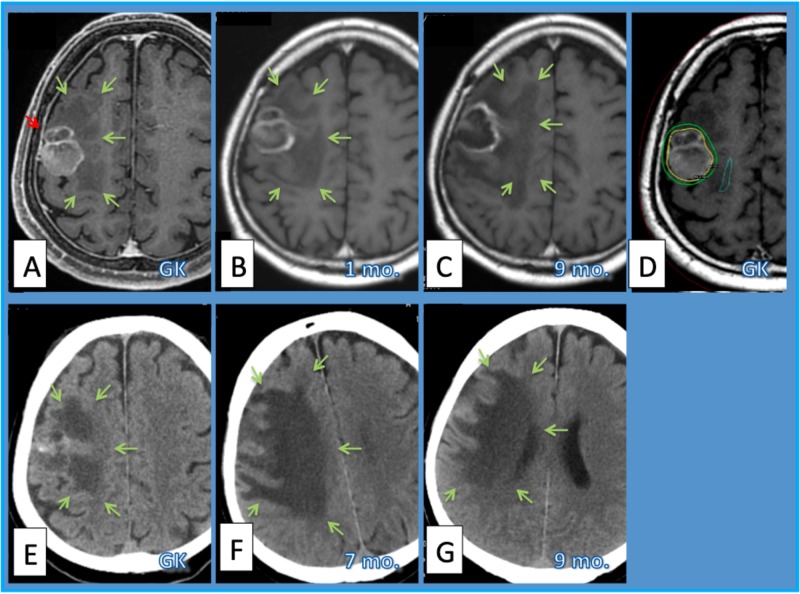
Case 6. Case 6.  Sixty-six-year-old man with lung adenocarcinoma brain metastasis.  Gd-enhanced MRI before GK (A) showed a right frontal mass lesion (red arrow) causing perifocal edema of low-intensity (green arrows). The volume of the tumor had been stable in MRI one month after GKRS (B) and in nine months after GKRS (C).  The wall of the cystic lesion became thinner within nine months after GKRS. The perifocal edema showed a peak on MRI seven months after GKRS (F) and was a little improved on CT nine months after GKRS (G). E: Plain CT before GKRS. Dose planning of GKRS (margin dose=20 Gy/ 2fx, PIV=12.9 ml) on GammaPlan (D). The yellow line shows the prescription isodose and the green line is the isodose line of 16 Gy/ 2 fx. One month after GKRS (C) MRI showed decreased central enhancement inside it, but the edematous area spread wider. Two months after GKRS enhanced volume became much smaller on iodine-enhanced computed tomography (D) and the edematous area improved a little. MRI = magnetic resonance imaging, GK=Gamma Knife, GKRS = GK radiosurgery, fx. = fraction
